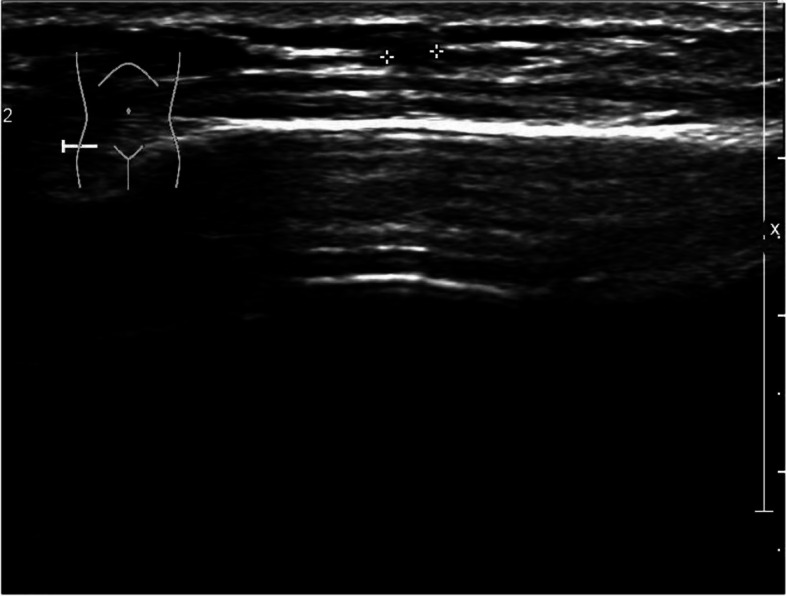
Superficial ultrasound reveals thrombus formation
